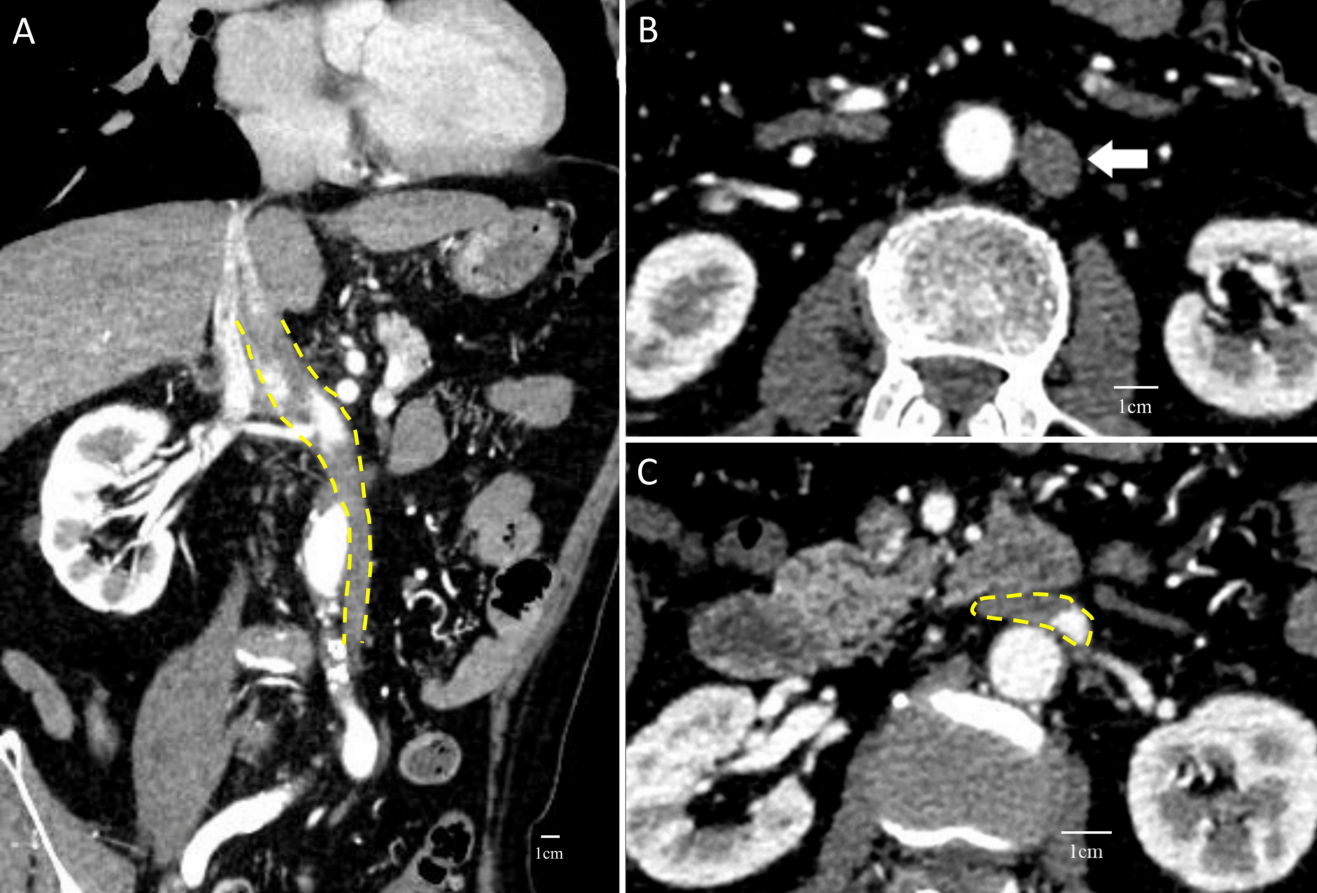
Left-sided IVC on enhanced CT. (A) Cross section including the IVC (yellow dashed line). (B) IVC (white arrow) on the left side of the aorta. (C) Crossing of the aorta and IVC (yellow dashed line) IVC: inferior vena cava; CT: computed tomography
